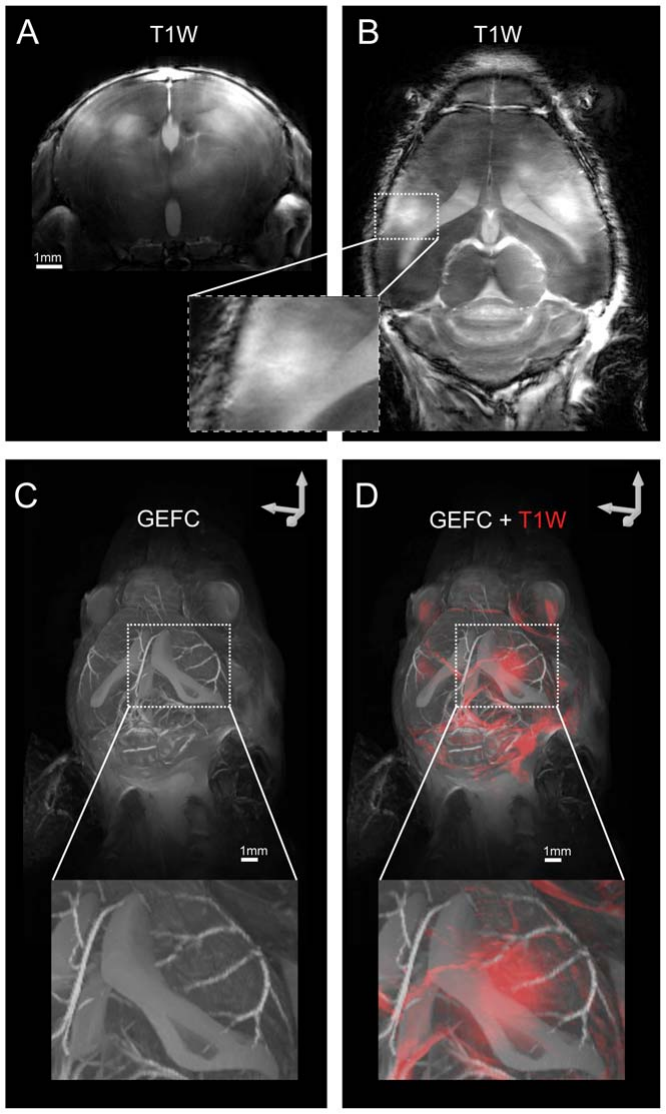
Three dimensional reconstruction of lesions in relation to the vascular and ventricular system. (A) Contrast-enhanced lesions (CEL) in coronal sections visualized by post Gd-DTPA contrast T1W MDEFT MRI (dotted line depicts horizontal slice in *B*). (B) CEL in horizontal sections imaged by post contrast T1W MDEFT (dotted line depicts coronal slice in *A*). (C) Maximum intensity projections (MIP) of images collected by 3D-GEFC showing the contrast-filled ventricular system and the cerebral vasculature (D) Overlay of a CEL 3D model (depicted in red) calculated from MIP of T1W MDEFT images on 3D ventricular and vascular system calculated from MIP of 3D-GEFC images.
